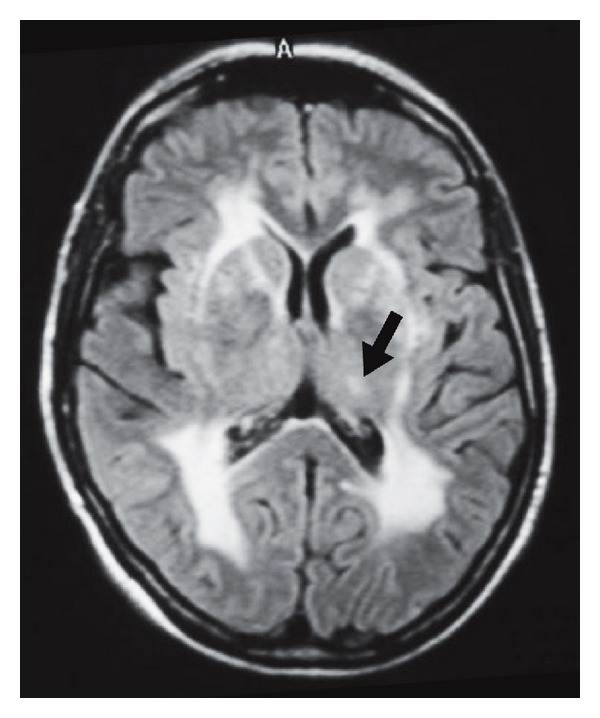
A patient with longstanding multiple sclerosis with diffuse leukoencephalopathy and a left thalamic demyelinating lesion (a) on FLAIR imaging.
